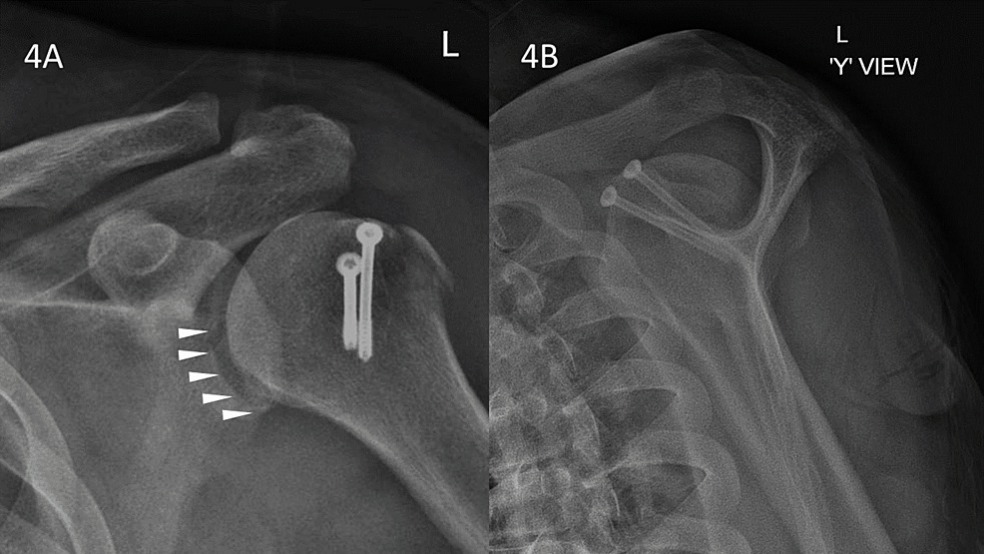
Check radiographs of the left shoulder. Plain radiographs (4A: anteroposterior and 4B: 'Y' view) showing the reduced greater tuberosity (GT) avulsion and the Bankart fragment secured in place with acceptable reduction (4A: white arrowheads).
